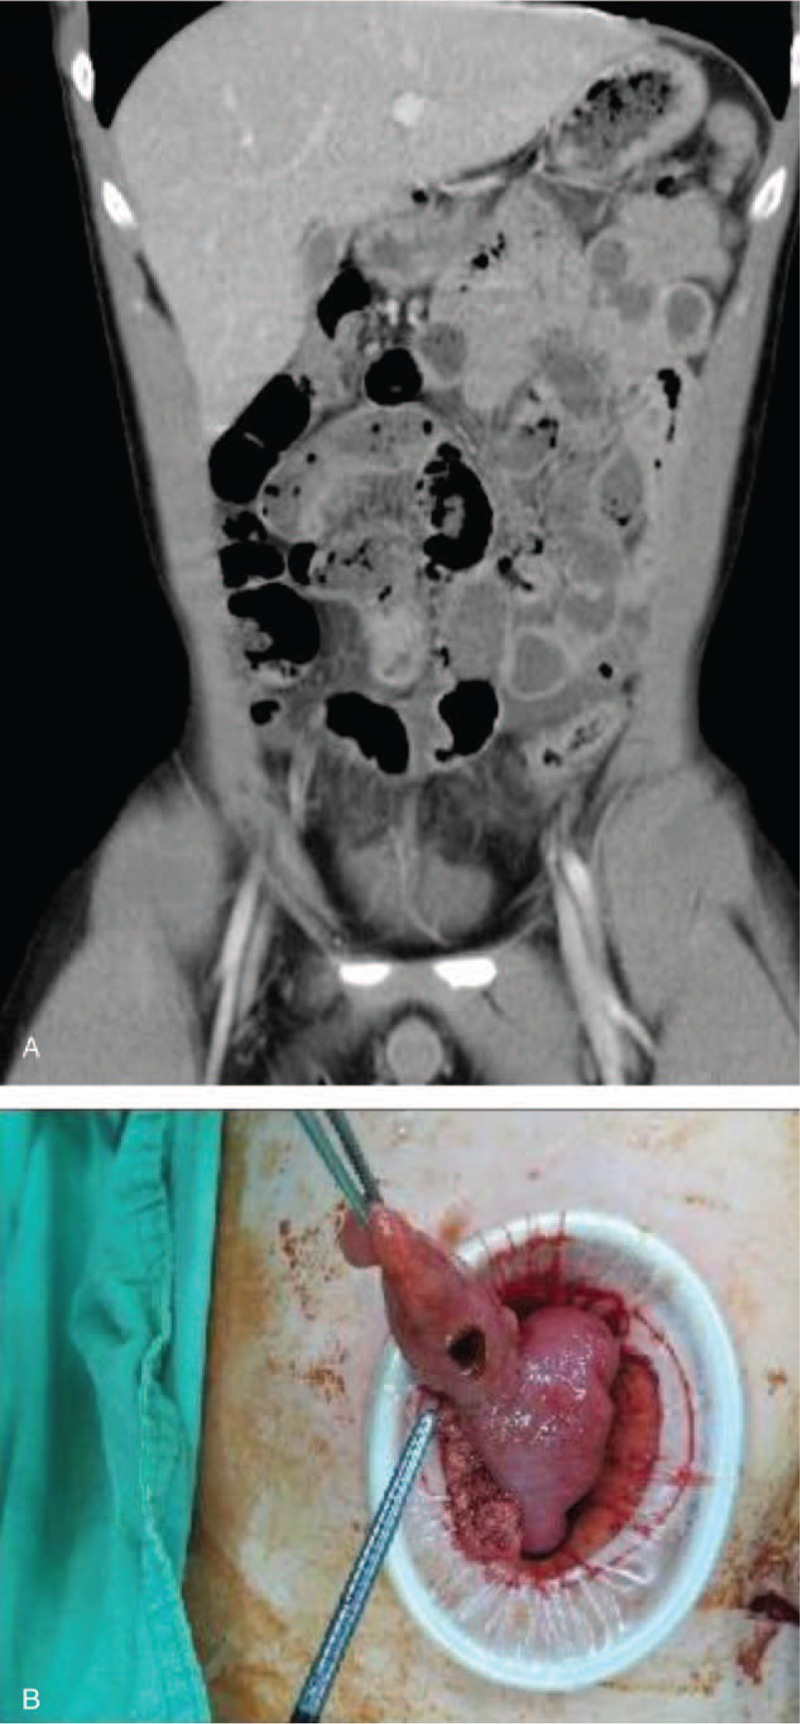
(A) Computed tomography showed suspect Meckel diverticulum in the distal ileum, (B) Meckel diverticulum with perforation, about 0.6 cm in diameter.
